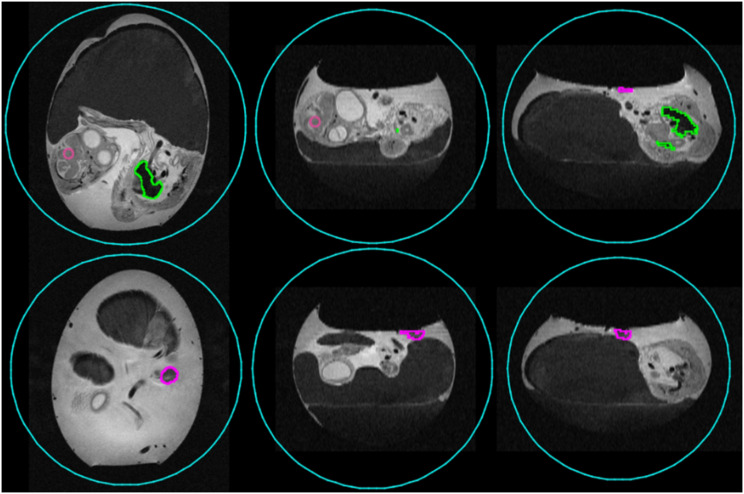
Example for VOI positioning. VOIs for liver (green) and xenograft (purple) were manually drawn based on the anatomical information from the T2-weighted TurboRARE scans (axial). A sphere (1 mm radius, rosa) was positioned in the center of the brain with the help of coregistered PET data as to mitigate spill-in effects. A sphere with a radius of 30 mm was placed around the whole egg to assess the total activity
